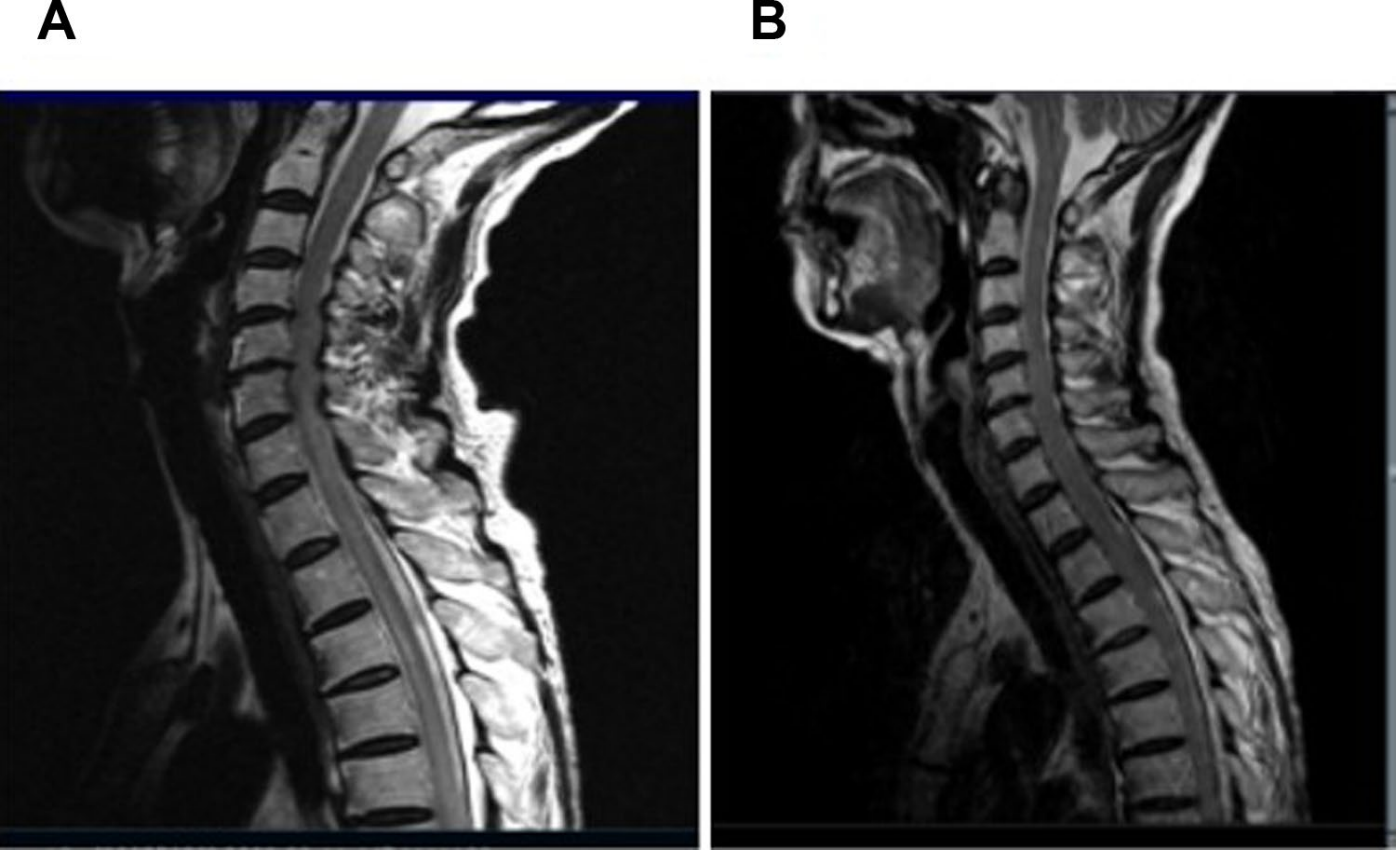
Immune injury of spinal cord was associated with durvalumab treatment. A Spinal magnetic resonance imaging (MRI) showed the swelling and hyperintensity of C7-T3 Segmentsspinal cord The lesions were laterally distributed in transverse section, and no enhancement was found in CE MRI. B Spinal MRI showed improvement after steroid pulse immunoglobulin and plasmapheresis therapy. The atrophy of the affected spinal cord were shown in the late images of follow-up
